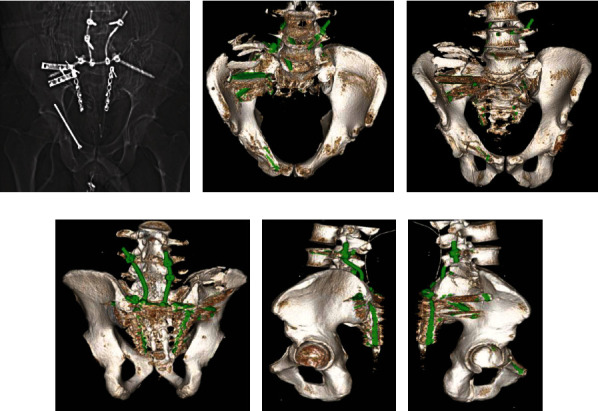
Postoperative radiological analysis. (a) Anteroposterior X-ray of the pelvis. (b)– (f) 3D-CT reconstruction showing the adequate reduction by lumbopelvic stabilization and the anterior acetabular column screw.
